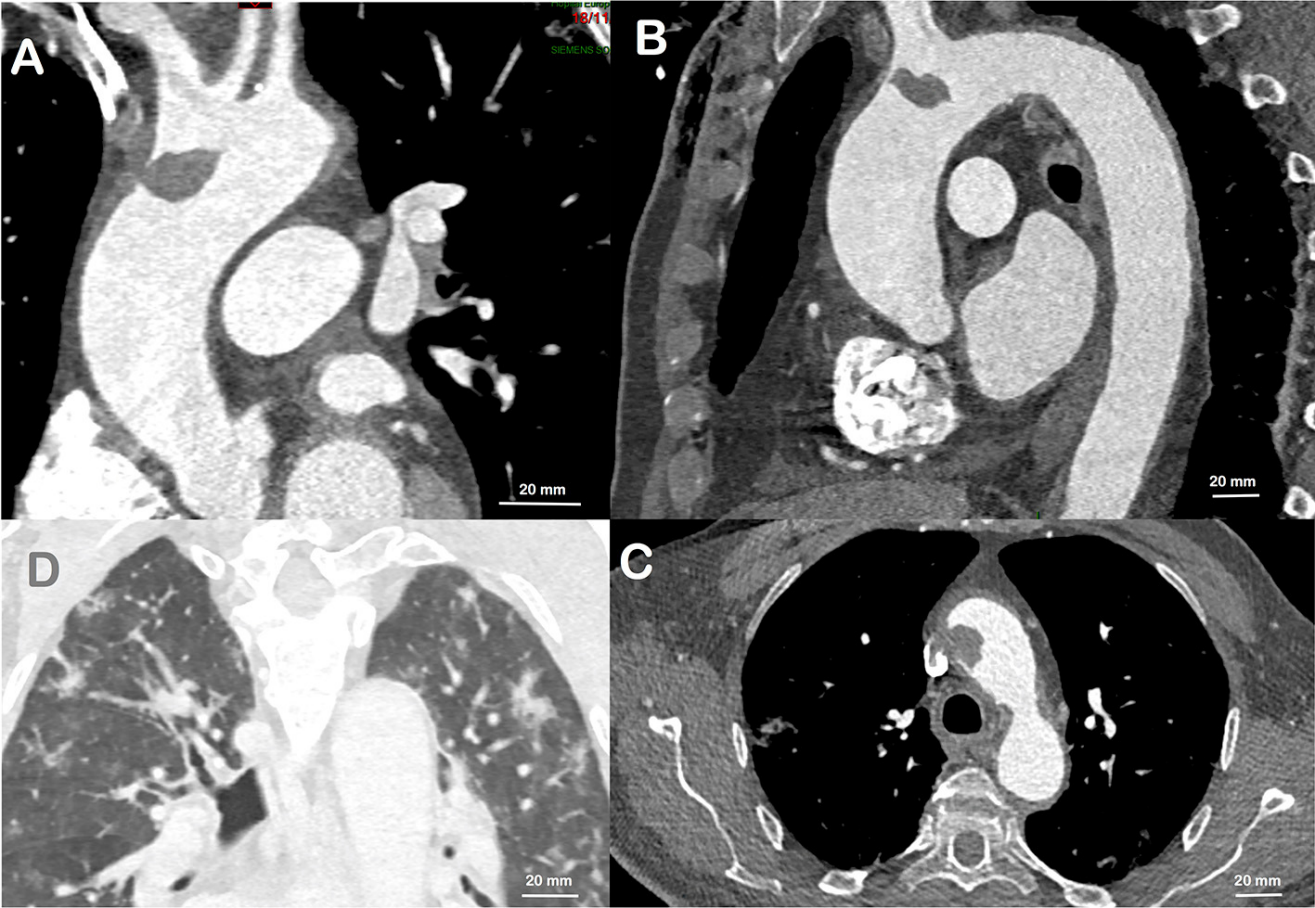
CT scan performed prior to cardiac surgery. A large thrombus at the base of the brachiocephalic trunk is visualized, and attached only to a short area on the aortic wall, in all views: sagittal (A), coronal (B), and transverse (C). Bilateral Ground-glass opacities of the lungs in relation with the COVID-19 (D).
